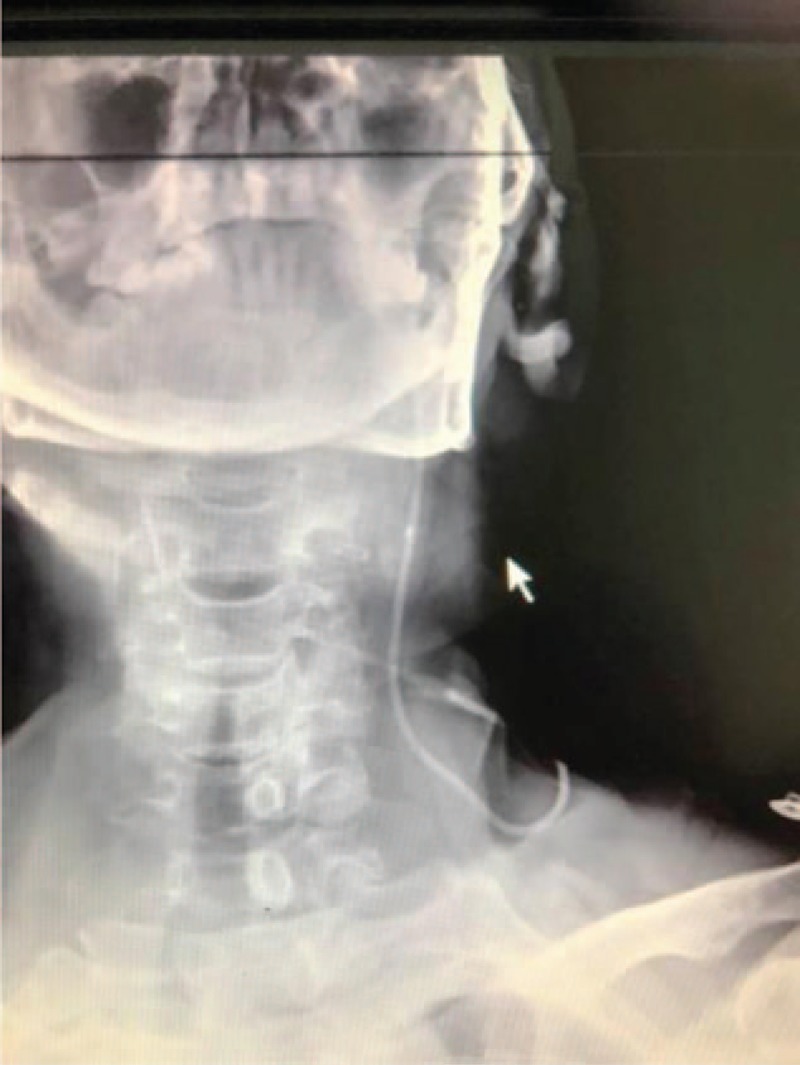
X-ray image of patient receiving ultrasound-guided retrograde puncture of internal jugular vein.
